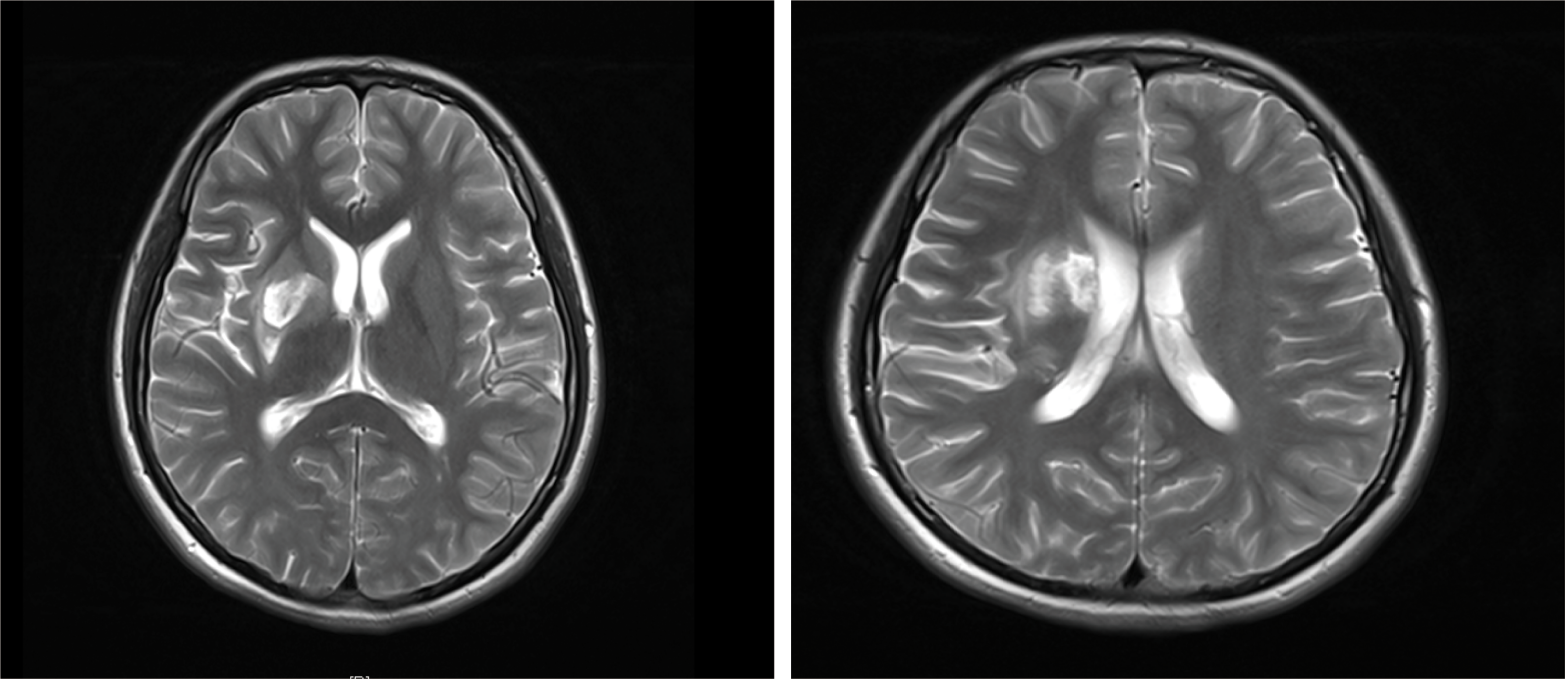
T2-weighted image of MRI: there are acute cerebral embolism lesions in multiple parts of the head.
